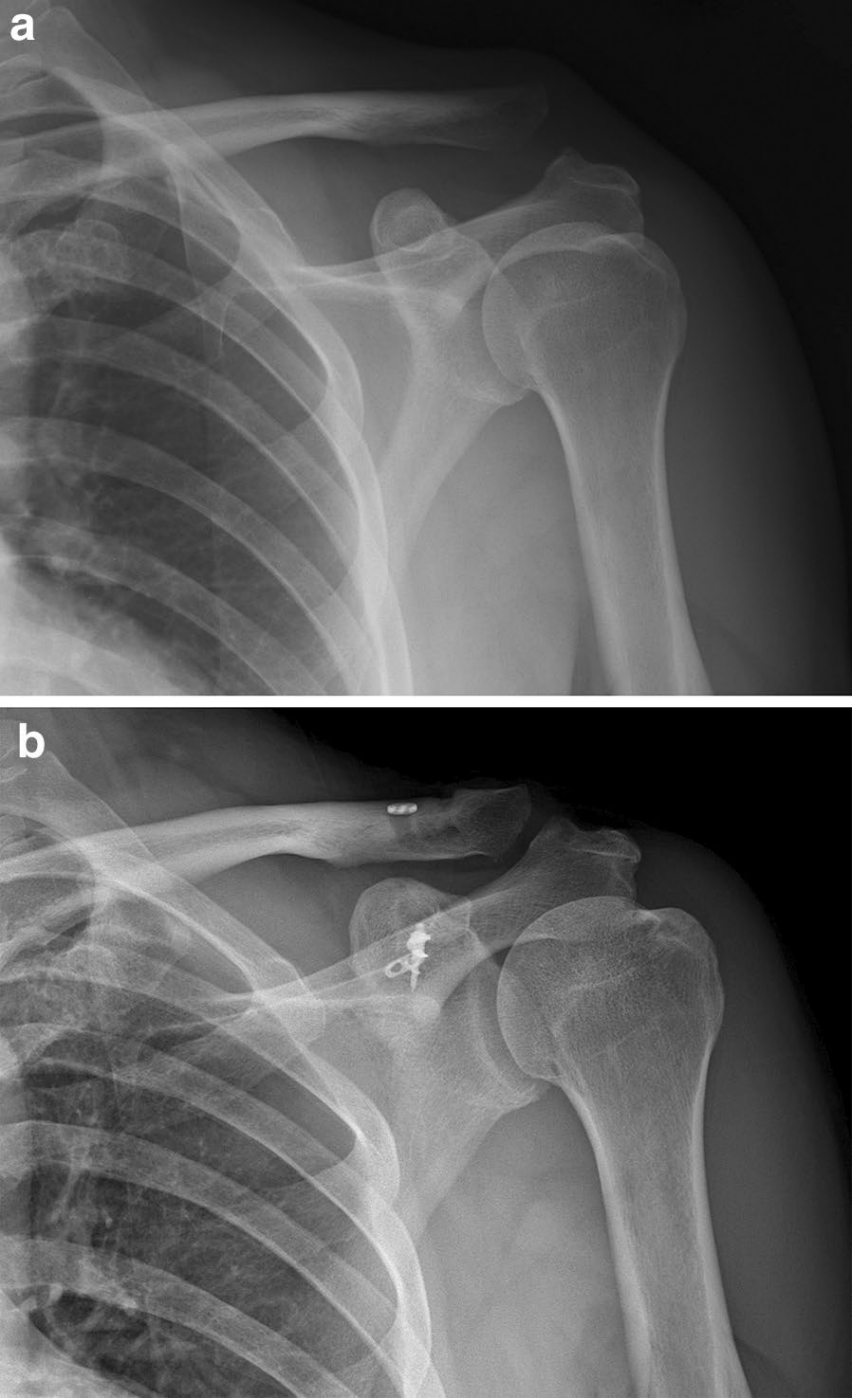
a Preoperative anteroposterior radiograph of Rockwood type V injury. b 67-months postoperative radiograph shows coracoclavicular interspace remained unchanged with an anchor and a double flip-button
